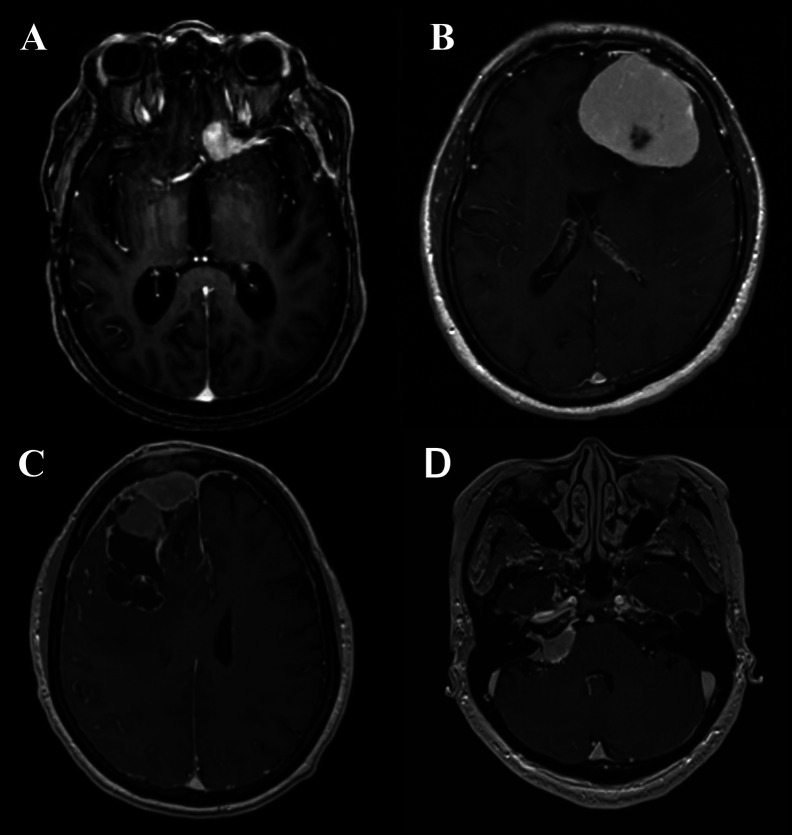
(A) Axial T1-post contrast MRI demonstrating an anterior clinoid meningioma with a characteristic dural tail. (B) Axial T1-post contrast MRI demonstrating a convexity meningioma with dural tails. (C) Axial T1-post contrast MRI demonstrating a meningioma with irregular edges abutting the superior sagittal sinus. (D) Axial T1-post contrast MRI demonstrating cerebellopontine angle meningioma.
